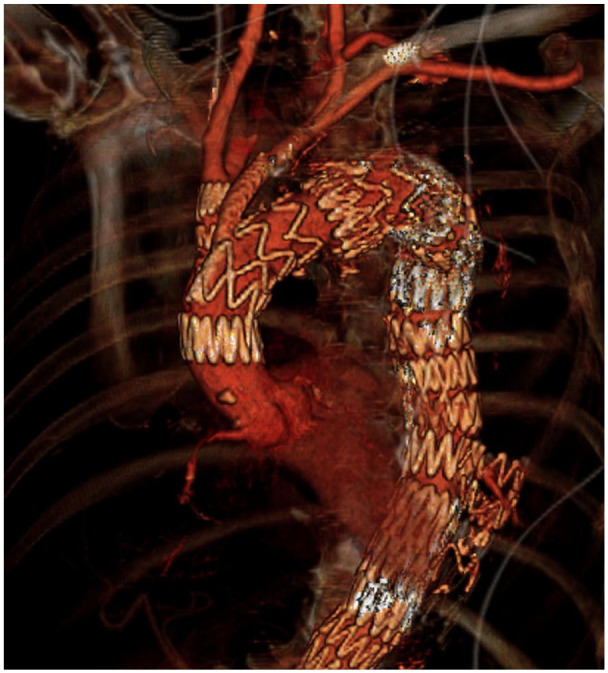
Three-dimensional reconstruction of the 1-month computed tomography angiography showing correct deployment of the endograft, patency of the branches and no signs of type I/III endoleak.
